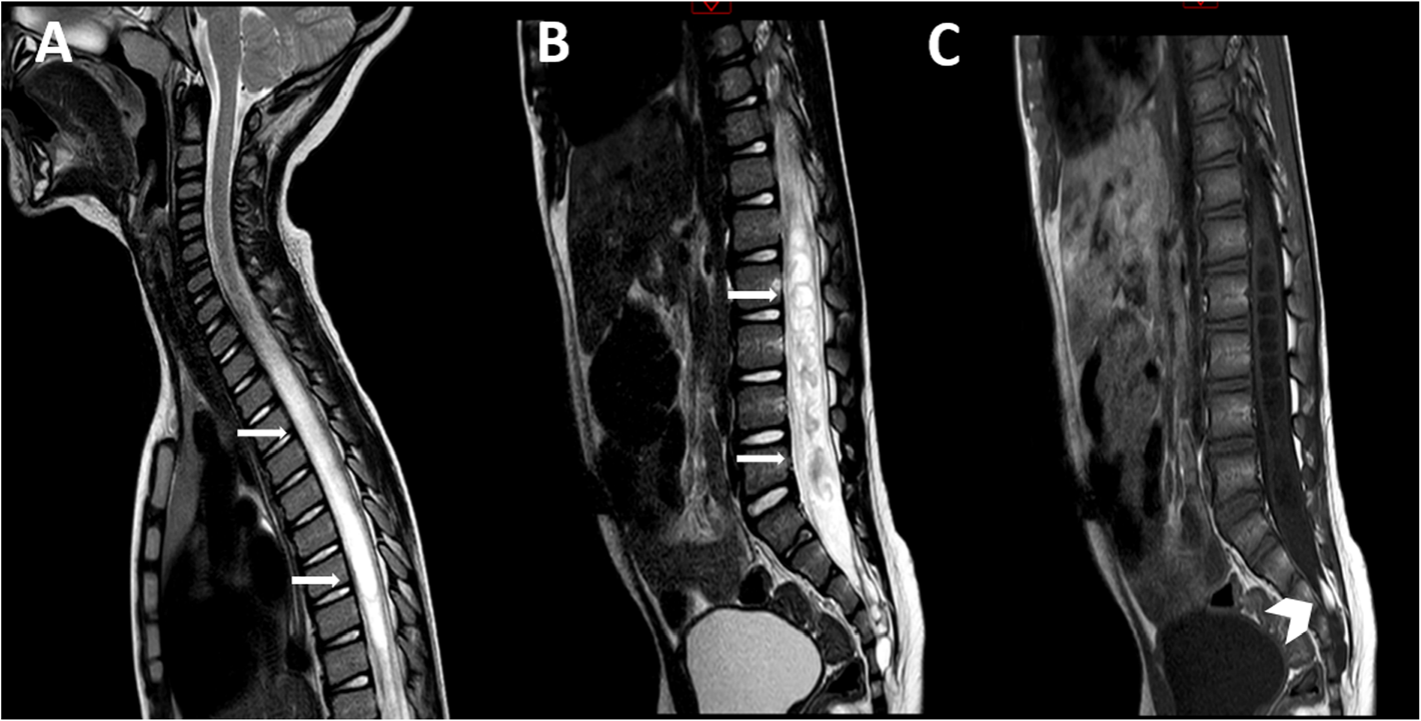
Sagittal T2 cervicodorsal (a) and lumbosacral MRI (b) of case #2 showing a very large dorsolumbar syringomyelia (arrows) and severe tethered cord, with the conus at S3-S4 level. The T1w sequence (c) demonstrates a small lipoma of the conus as a cause of tethering (head arrow)
